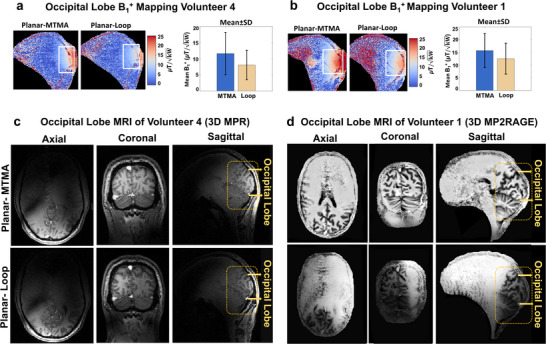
Comparison of the transmit field B₁^+^ mapping and structural MRI of the occipital lobe of the brain using the Planar‐MTMA and Planar‐Loop configuration in two healthy male volunteers. (a, b) Transmit field B₁^+^ maps (sagittal slice) obtained from volunteers 4 and 1 covering the occipital lobe of the brain using the Planar‐MTMA and the Planar‐Loop. The bar plot shows that the Planar‐MTMA yielded a stronger B₁^+^ than the Planar‐Loop for the ROI delineated by the white rectangle. (c) Sagittal, axial, and coronal T_1_‐weighted images of the occipital lobe derived from 3D MPR MRI of volunteer 4 and (d) 3D MP2RAGE MRI of volunteer 1 using the Planar‐MTMA and Planar‐Loop. Yellow dashed boxes denote the occipital lobe region of the brain. The Planar‐MTMA improved signal coverage at greater depths, enhancing the visibility of posterior brain structures, including the cerebellum. In contrast, images acquired with the Planar‐Loop exhibited reduced signal intensity and more limited coverage in deeper brain regions.
